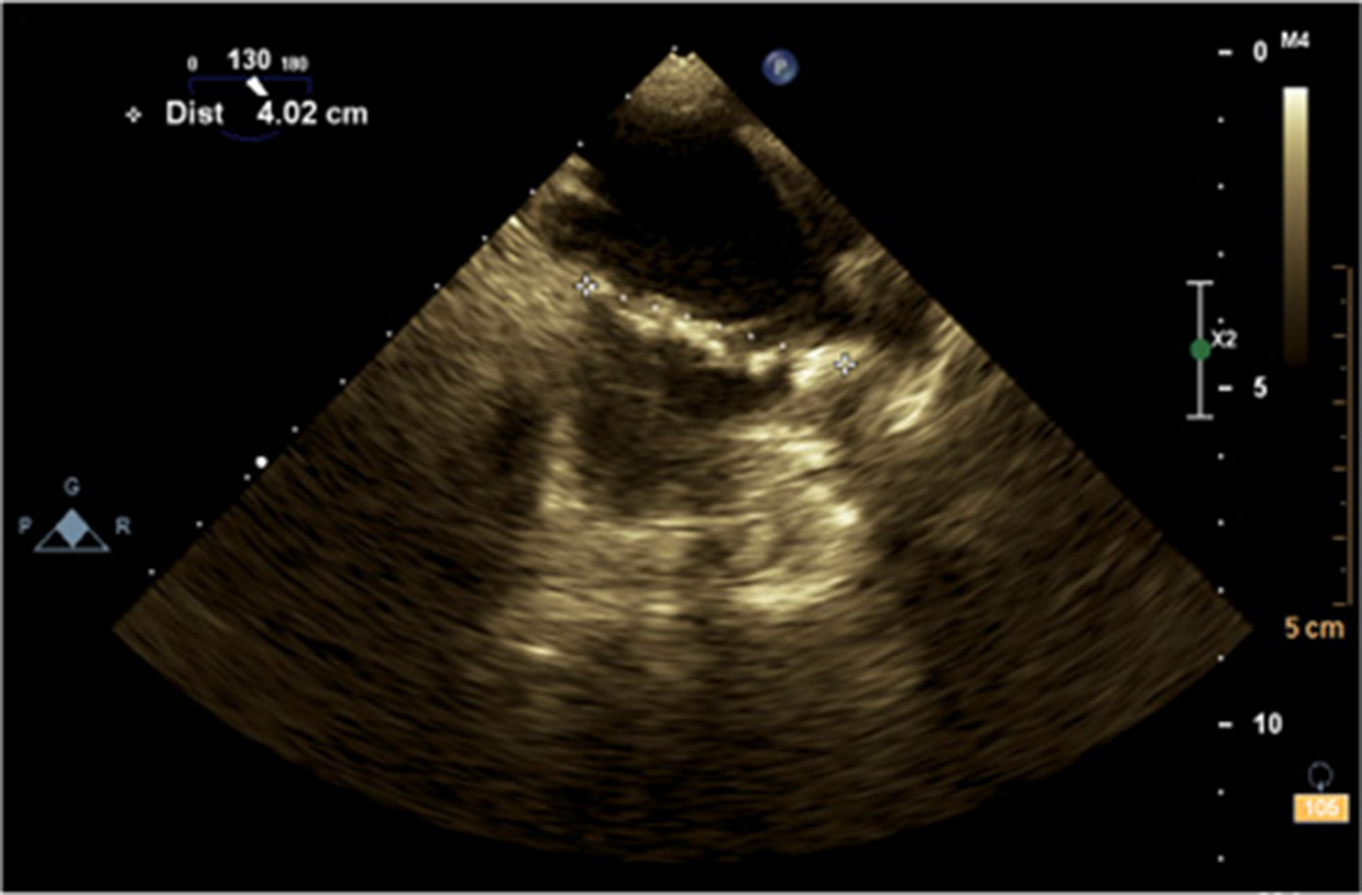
The transesophageal echocardiography in the midesophageal 130° view shows an elongated (4 cm in length) irregular hypermobile echodense mass in the right atrium.
